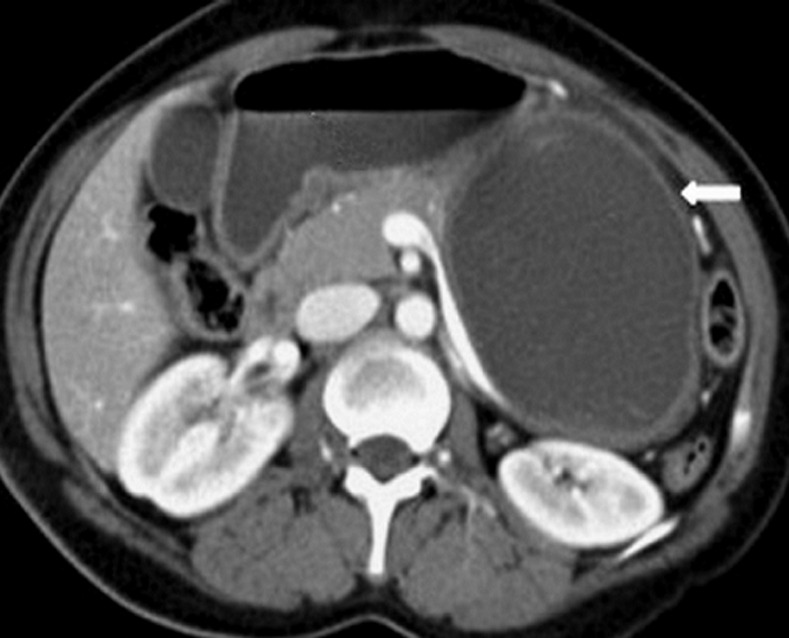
computed tomography scan of the hydatid cyst of the tail of the pancreas; large cystic mass (white thick arrow) measuring 11.5 cm, located in the tail of the pancreas
